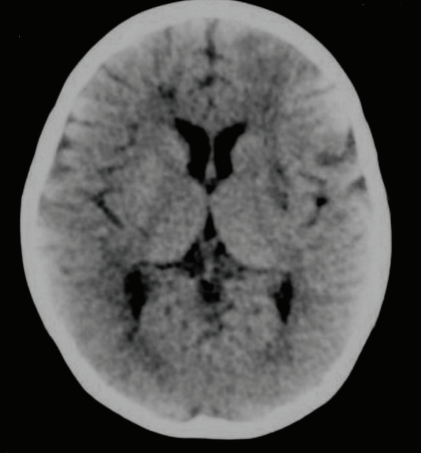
Axial brain CT scan showing no significant lesion.
